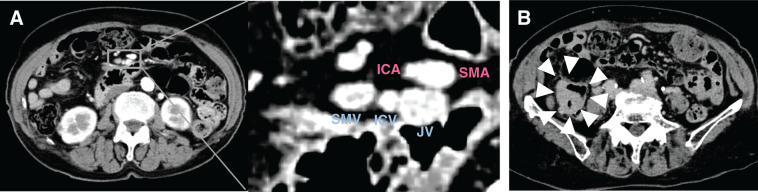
Contrast-enhanced abdominal CT findings. (A) CT shows ICV flows into the JV. (B) The arrowheads indicate the tumor in the cecum. ICA, ileocolic vein; ICV, ileocolic vein; JV, jejunal vein; SMA, superior mesenteric artery; SMV, superior mesenteric vein
